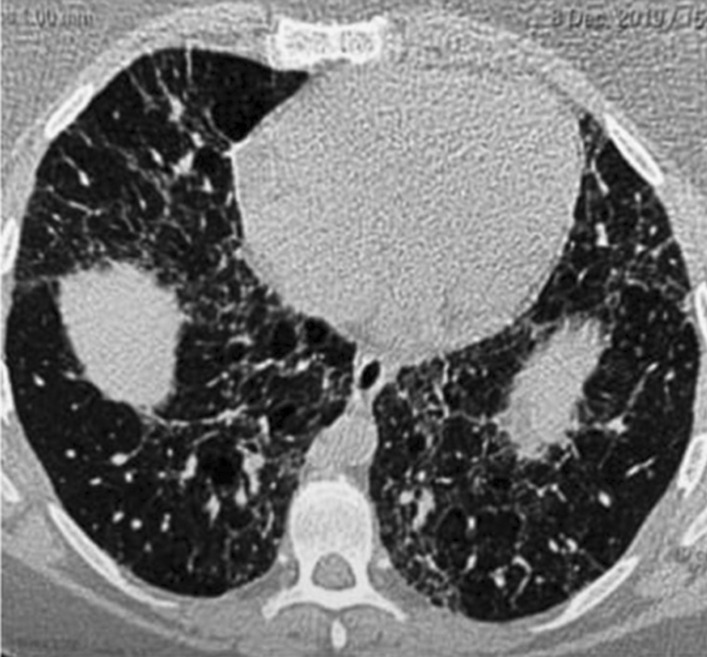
Female patients 41 years with fibrotic HP, intermediate probability of PH in echocardiography and severe restriction
